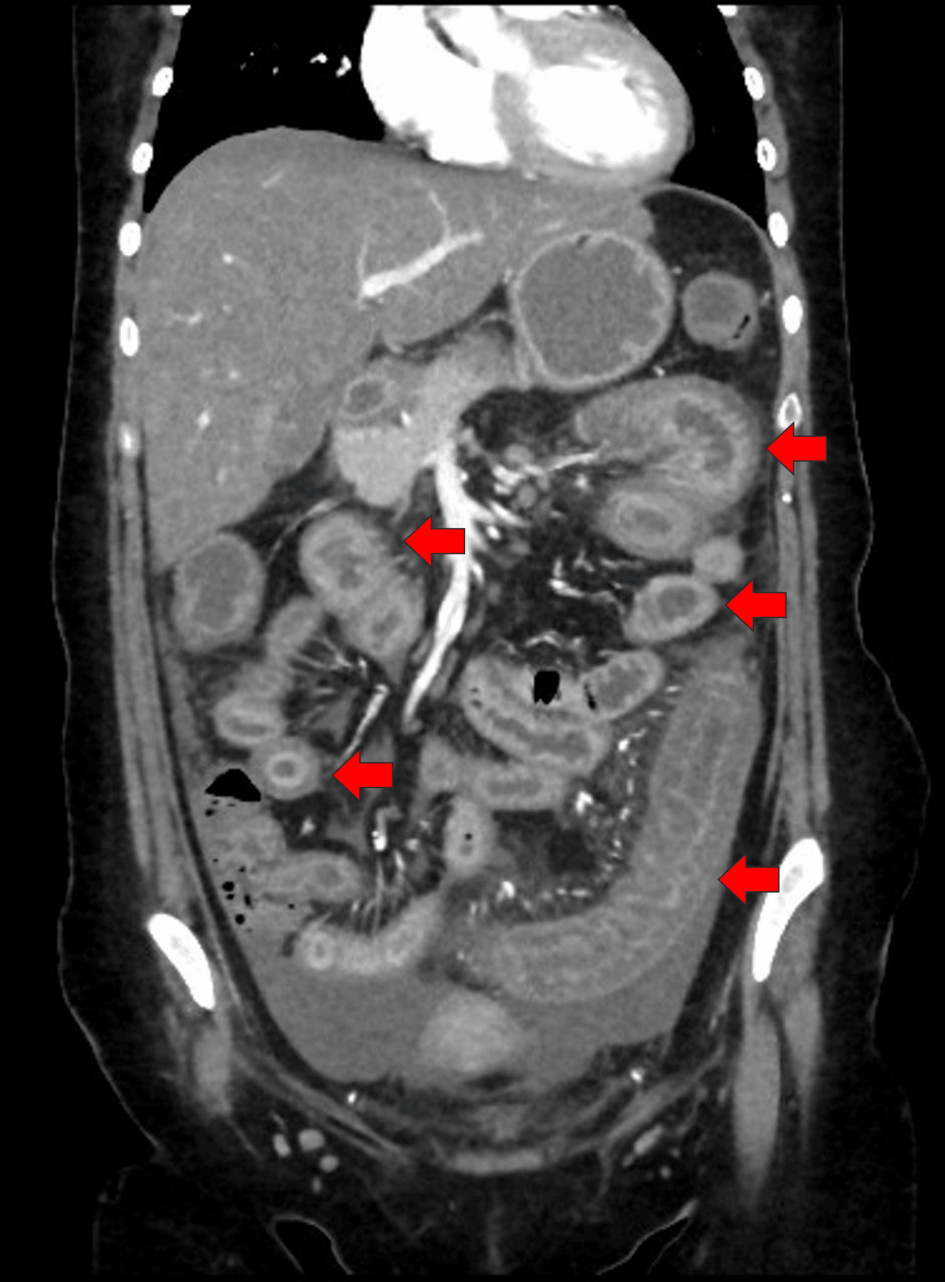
Abdominal CT scan (coronal view) Descending and sigmoid colon, and the rectum walls were thickened and edematous (red arrows); significant pericolonic fat stranding and free fluid with preserved enhancement suggested an active systemic inflammatory process.
